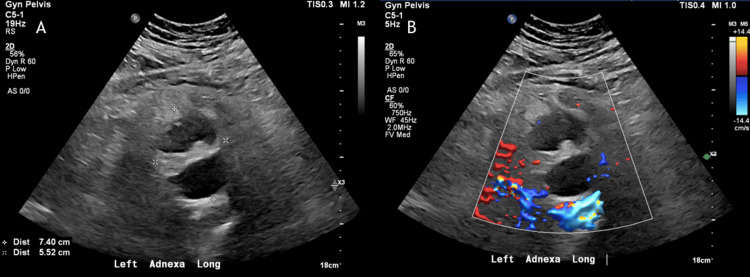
Gray-scale and doppler pelvic ultrasound demonstrating the previously seen lesion on computed tomography (CT) (1A) Gray-scale ultrasound, (1B) Doppler ultrasound Complex left adnexal lesion with multiple anechoic cystic-like lesions with trace internal echoes, thick hyperechoic septations, and surrounding hyperemia measuring up to 7.4 x 5.5 cm may reflect tubo-ovarian abscess.
